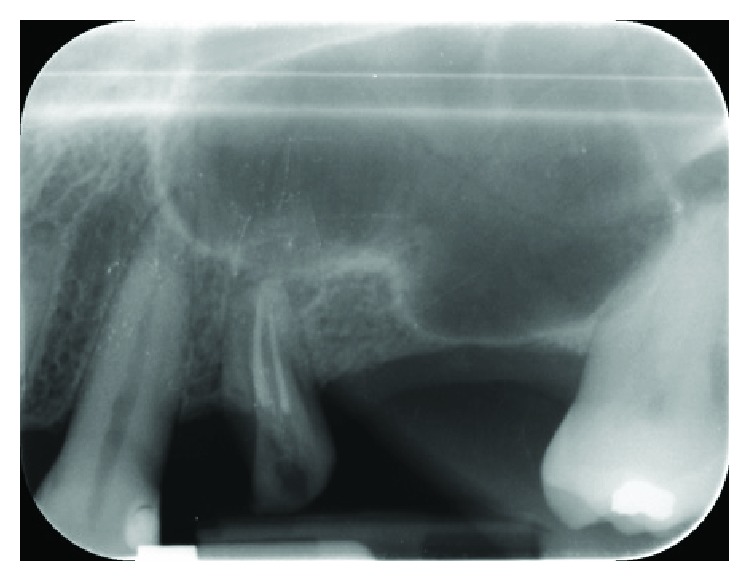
Intraoral radiograph (Rinn® collimator) of the surgery site.
